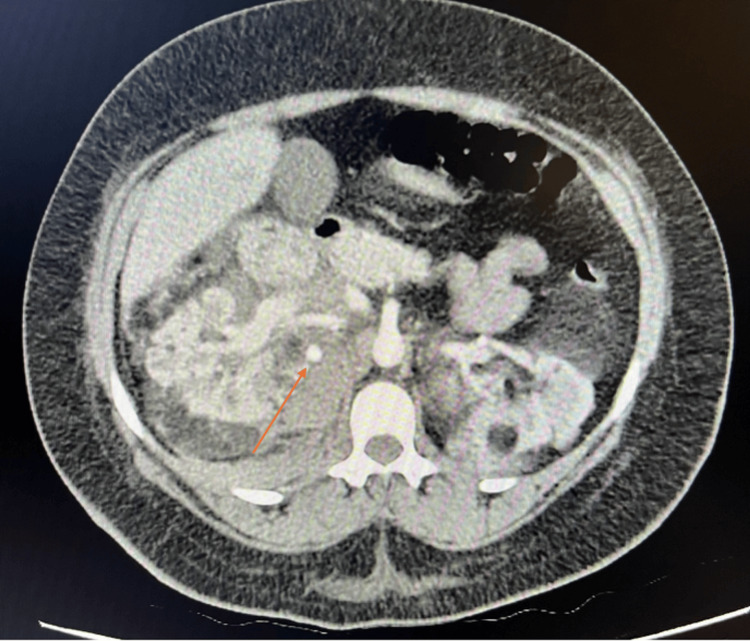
Index CT kidney triple phase: arterial phase with axial views of the abdomen The arrow highlights the right renal artery pseudoaneurysm as a hyperdense circular abnormality.
